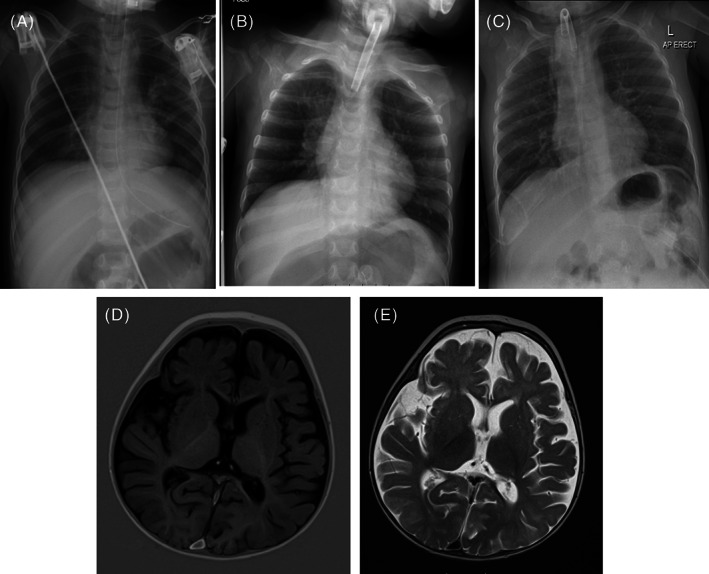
Imaging from Case 1. Chest X‐rays before (A), 3 months (B) and 11 months (C) after initiation of ventilatory support. (A) Both diaphragms are flattened and at equal levels suggesting abnormal dynamics of the diaphragms. (B) The right hemi‐diaphragm is ascended with tracheostomy in situ. (C) The diaphragms look in physiological position with tracheostomy in situ. Brain MRI T1 (D) and T2 (E) sequences showing cerebral atrophy and delayed myelination predominant in the frontal lobes at 11 months of age.
